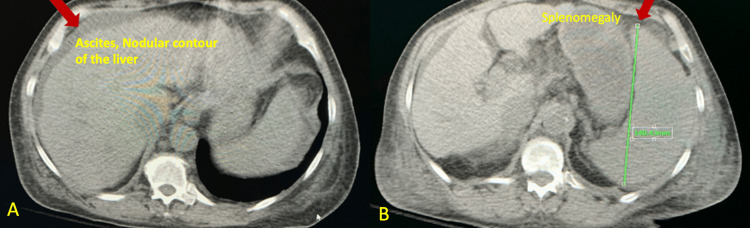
CT scan of the abdomen/pelvis - (A) Ascites and the nodular contour of the liver (B) Splenomegaly
